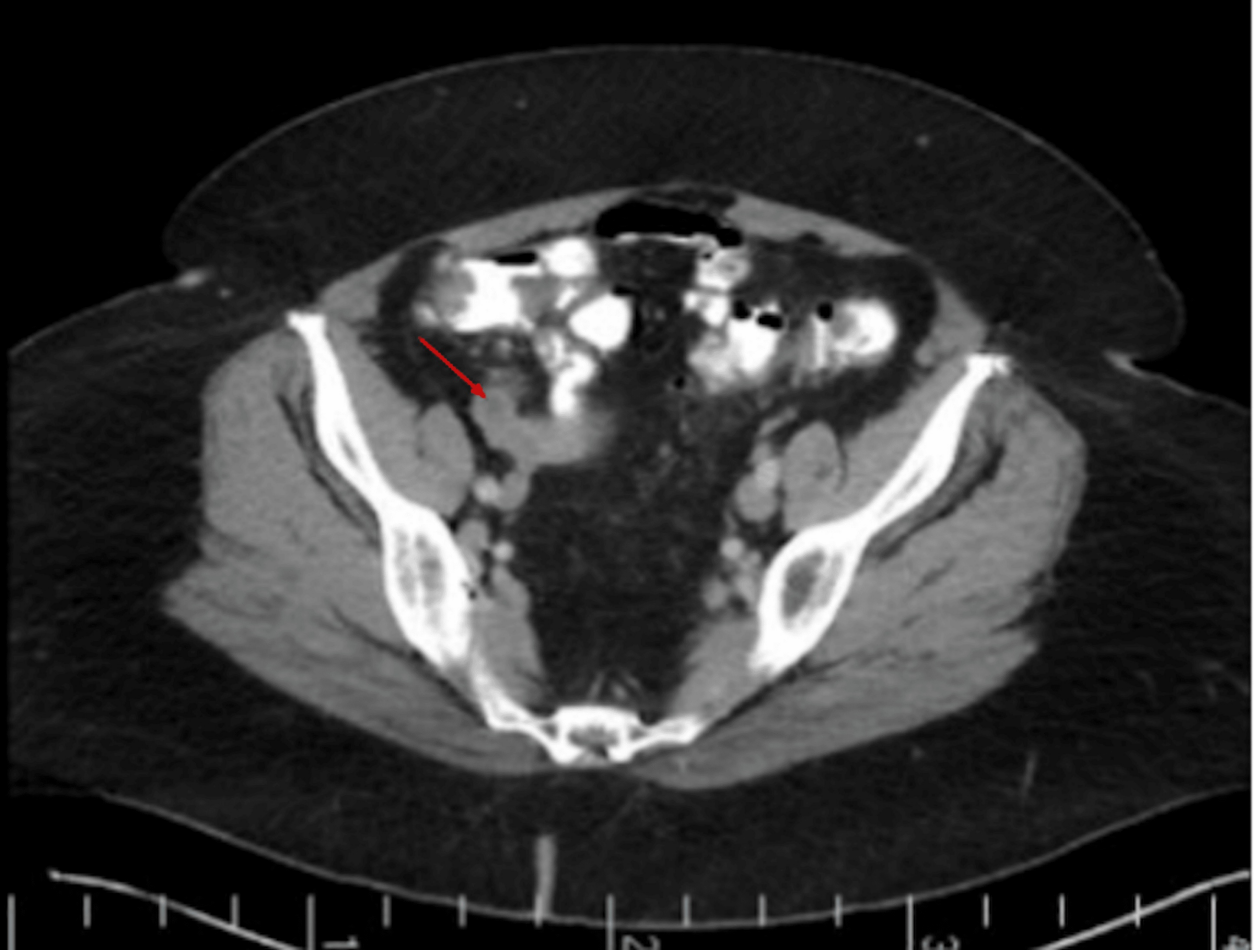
Axial CT image at the level of the pelvis; the red arrow demonstrates a round cystic lesion that originated from the cecum (post-colonoscopy, 2024). No associated nodularity and no evidence of rupture.
